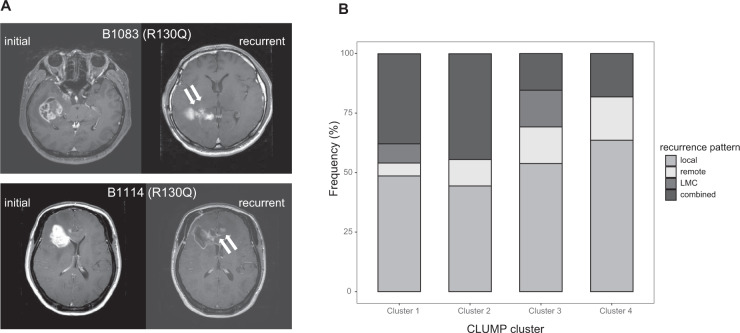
Radiographic failure patterns of GBM tumors with *PTEN* edge mutations. A Representative MR images depicting the locally invasive failure pattern of GBM tumors with *PTEN* edge mutation. Two GBM patients with *PTEN* edge mutation R130Q demonstrated locally invasive failure patterns, with tumors transmitted to the contralateral hemisphere in a locally invasive, infiltrative manner. B Distribution of radiographic recurrence patterns in CLUM-defined clusters. Edge mutations were included in cluster 4, while, the nuclear mutation was included in cluster 1 with other mutations in the C2 domain. LMC, leptomeningeal seeding.
